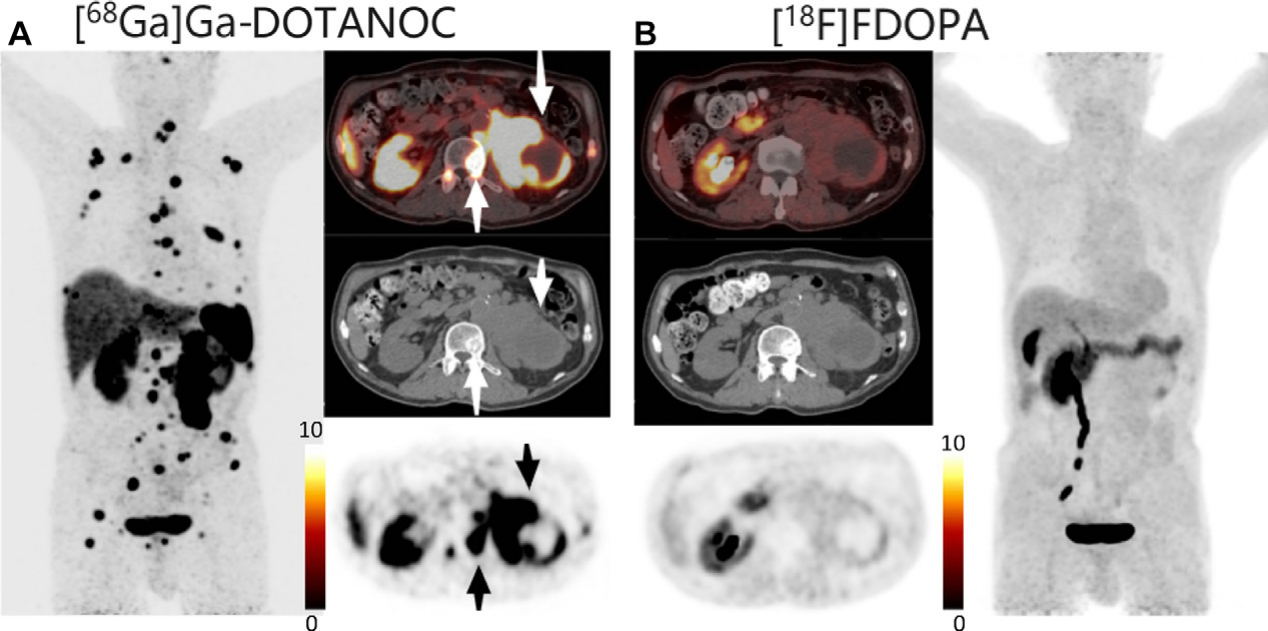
PET/CT scans in a 37-year-old male patient with *SDHx*
